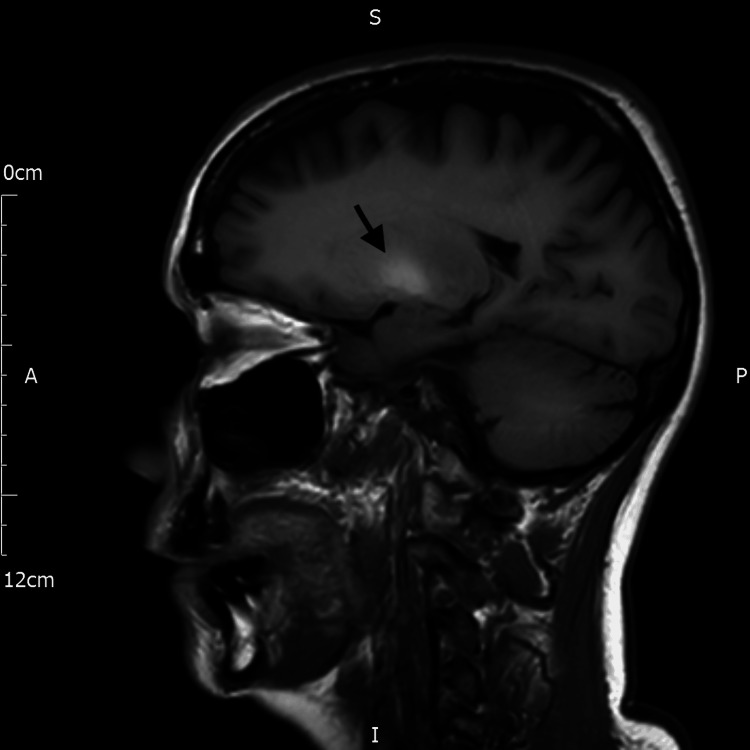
T1-weighted sagittal MRI of the brain The arrow indicates high signal intensity in the region of the basal ganglia
